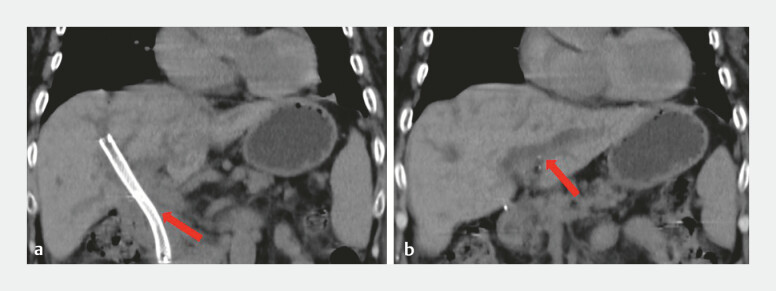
CT imaging prior to ERCP.
